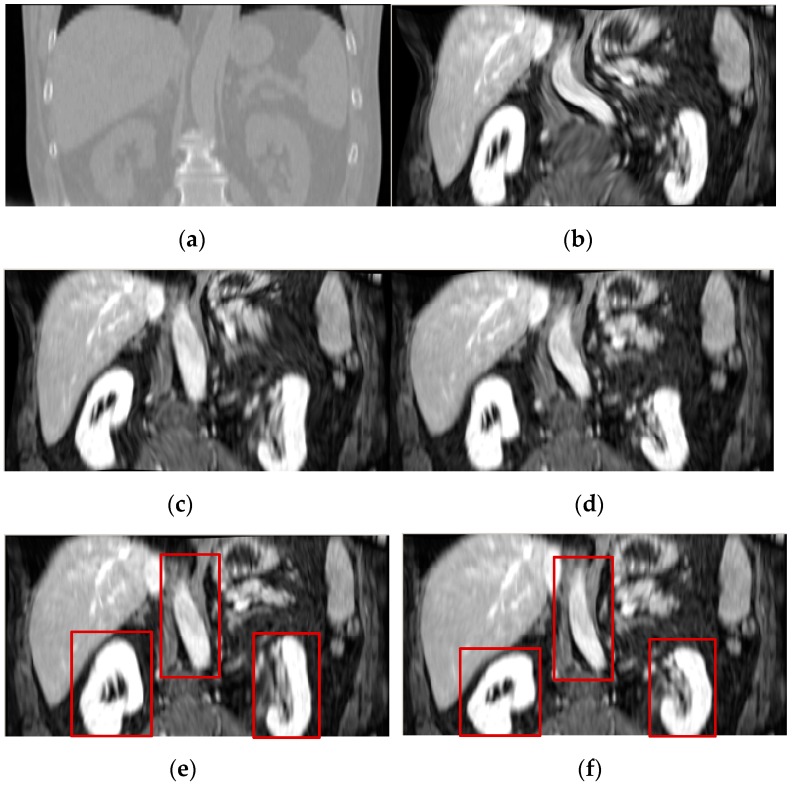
The registration results of all evaluated methods operating on 3D CT–MR images. (a) CT image (reference image); (b) MR image (float image); (c) ESSD; (d) MIND; (e) HLCSO; and, (f) FMIND.
